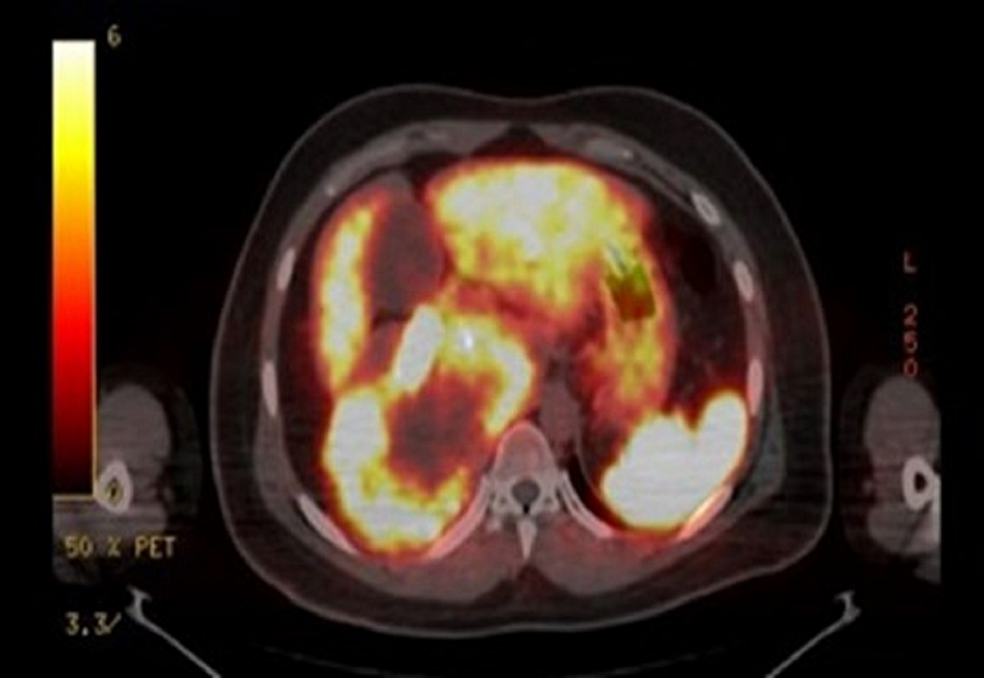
PET-CT scan showing radiogallium heterogeneously avid, right retroperitoneal hypervascular mass, with central necrosis and multiple foci of cystic changes. There is gross interval stability in the mass size and persistent non-visualization of the right kidney. PET: Positron emission tomography.
